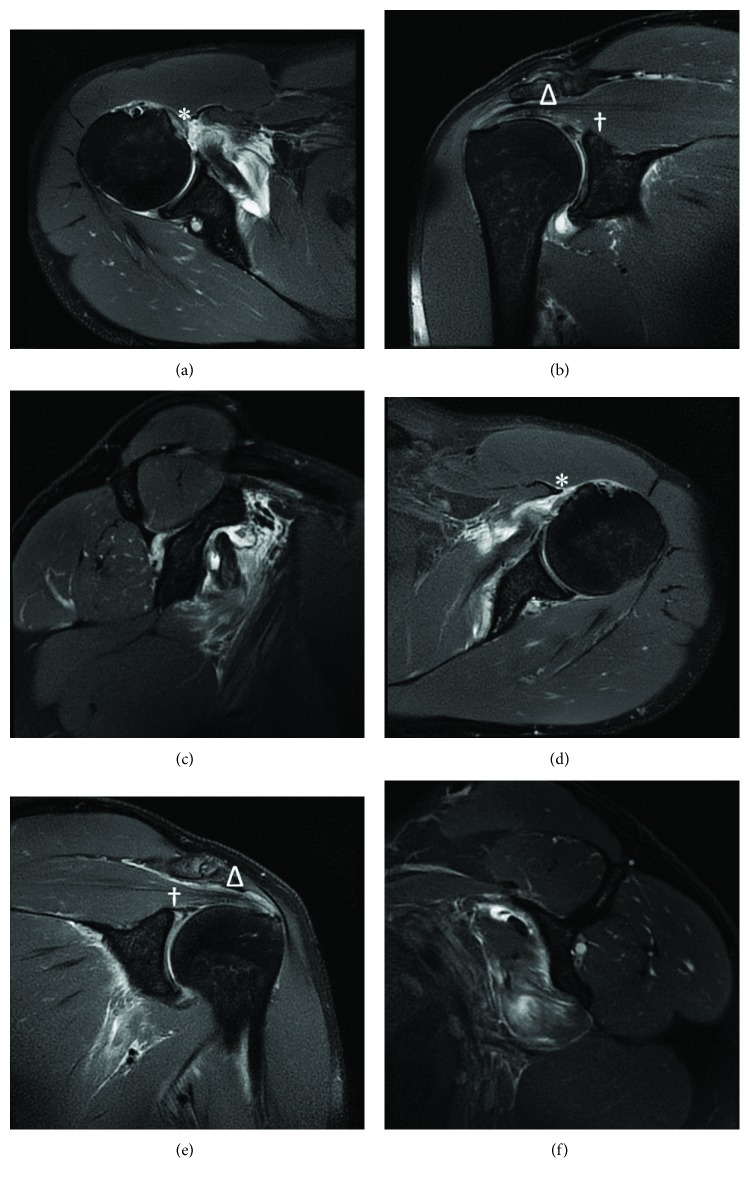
Noncontrast MRI of (a) axial, (b) coronal, and (c) sagittal views of the right shoulder; (d) axial, (e) coronal, and (f) sagittal views of the left shoulder. ∗ denotes a torn subscapularis tendon. Δ denotes an intact supraspinatus tendon. † denotes a type 4 SLAP tear. Sagittal views demonstrate edematous changes and retraction, without significant fatty infiltration.
